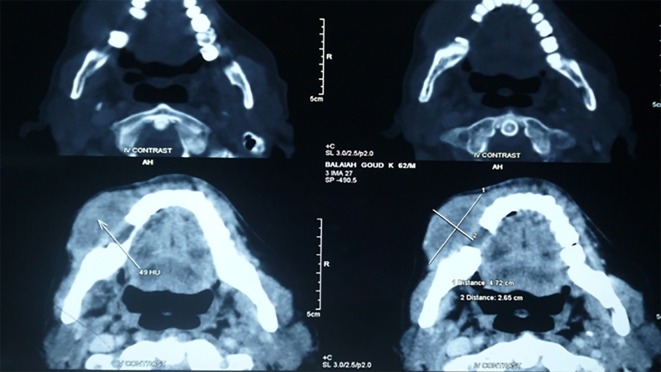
CT scan showing extensive lytic lesion involving the ramus and condyle with destruction of inferior border of mandible.
